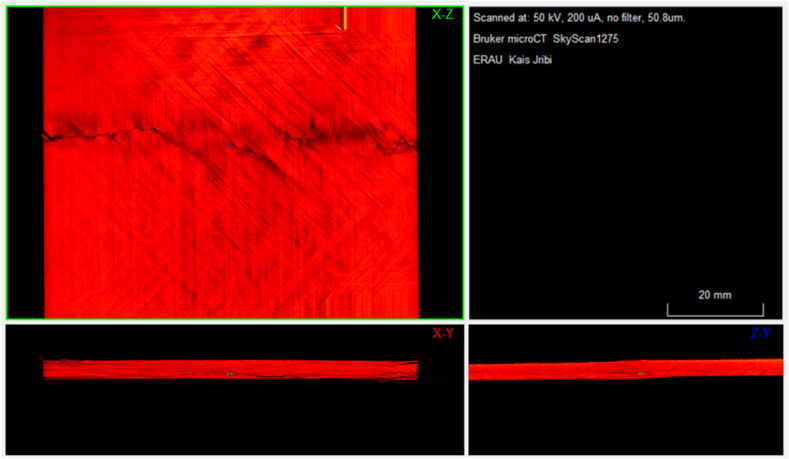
3D projection of the final damage topology from CT Scan.
